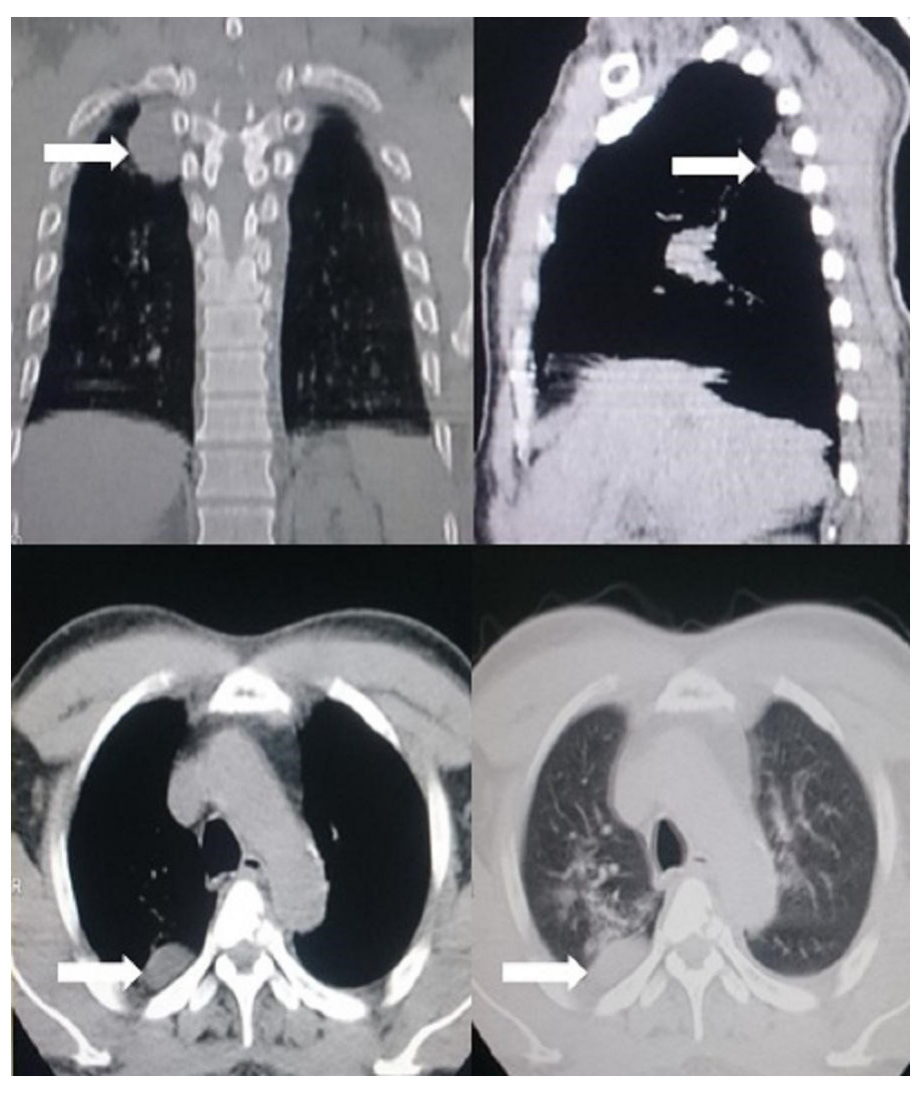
CT images revealing presence of fibrotic changes along with a pleural based nodule in the right lung.
